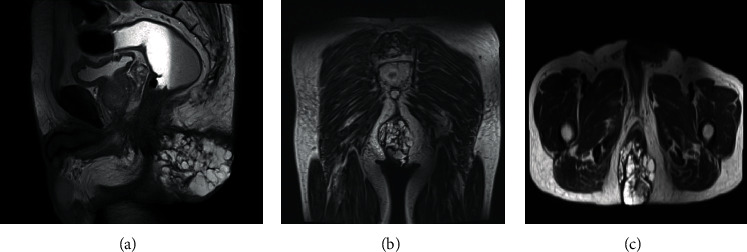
(a–c) MRI of the pelvis showing large mass involving the anal sphincter.
